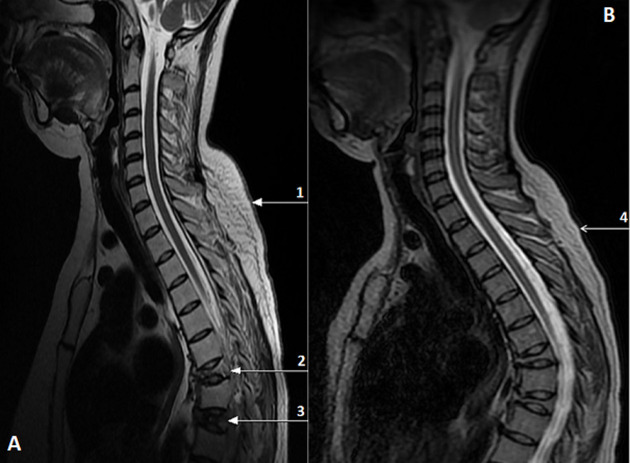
axial chest MRI T2-weighted before (A) and one year after surgery (B) reveled buffalo neck (arrow 1 and 4) and vertebral compression fractures of D7 and D9 (arrow 2 and 3)
